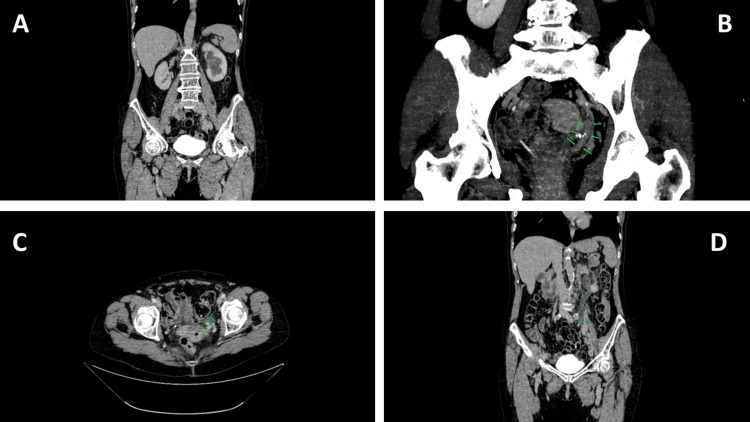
CT imaging demonstrating a ureteral tumor (A) Coronal CT image in the excretory phase showing dilatation of the left renal calyces. (B) Contrast-enhanced coronal CT image (maximum intensity projection) demonstrating a soft-tissue mass within the pelvic ureter (arrows). (C) Contrast-enhanced axial CT image in the nephrographic phase showing an enhancing soft-tissue mass within the left ureter (arrows). (D) Contrast-enhanced coronal CT image demonstrating a soft-tissue mass within the pelvic ureter (arrows).
